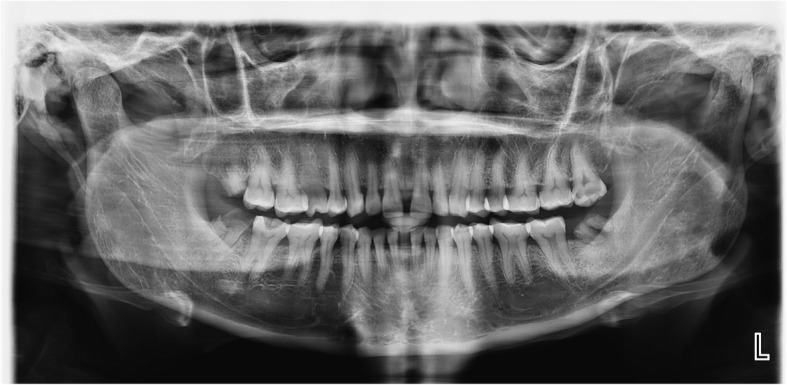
Orthopantomogram with multiple oral cavities
